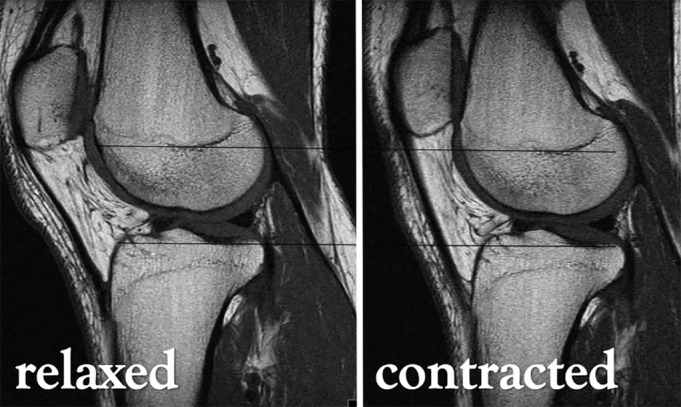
Proximal migration of the patella between conventional (left) and quadriceps contracted (right) sagittal MRI sequences, from Barroso *et al*.^26^.
